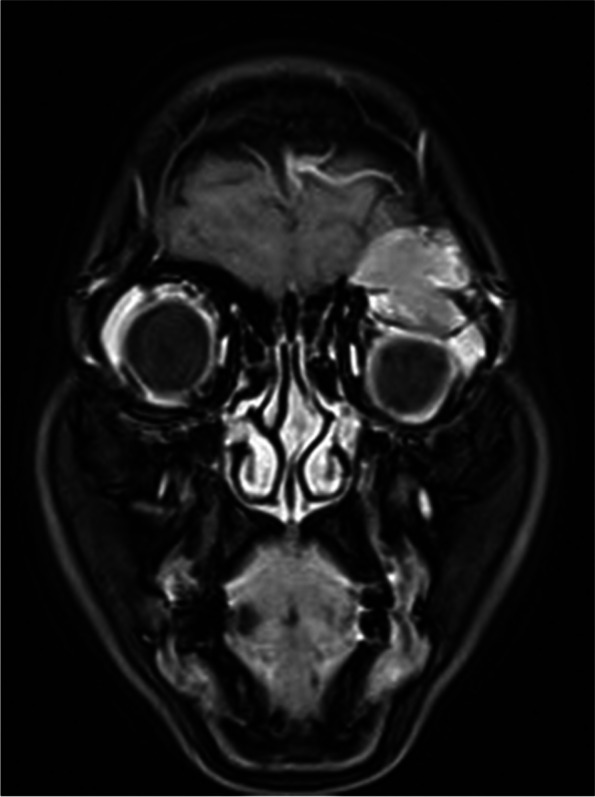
Enhanced magnetic resonance imaging showed a non-enhancing mass that presented hyperintense
